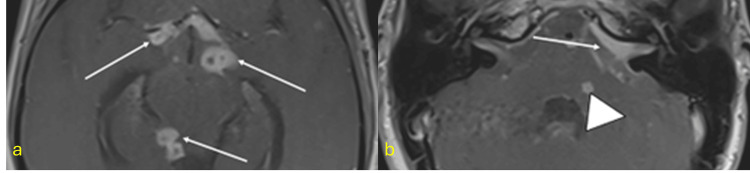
CNS tuberculosis ADA: adenosine deaminase; MRI: magnetic resonance imaging; CNS: central nervous system Axial T1-weighted post-contrast MRI images showing (a) nodular leptomeningeal enhancement in the basal cisterns (arrows) and (b) a tuberculoma in the left cerebellopontine angle (arrow) and a small tuberculoma within the cerebellar parenchyma (arrowhead). Diagnosis confirmed by cerebrospinal fluid (CSF) analysis showing lymphocytic pleocytosis, elevated ADA, and positive GeneXpert MTB/RIF
